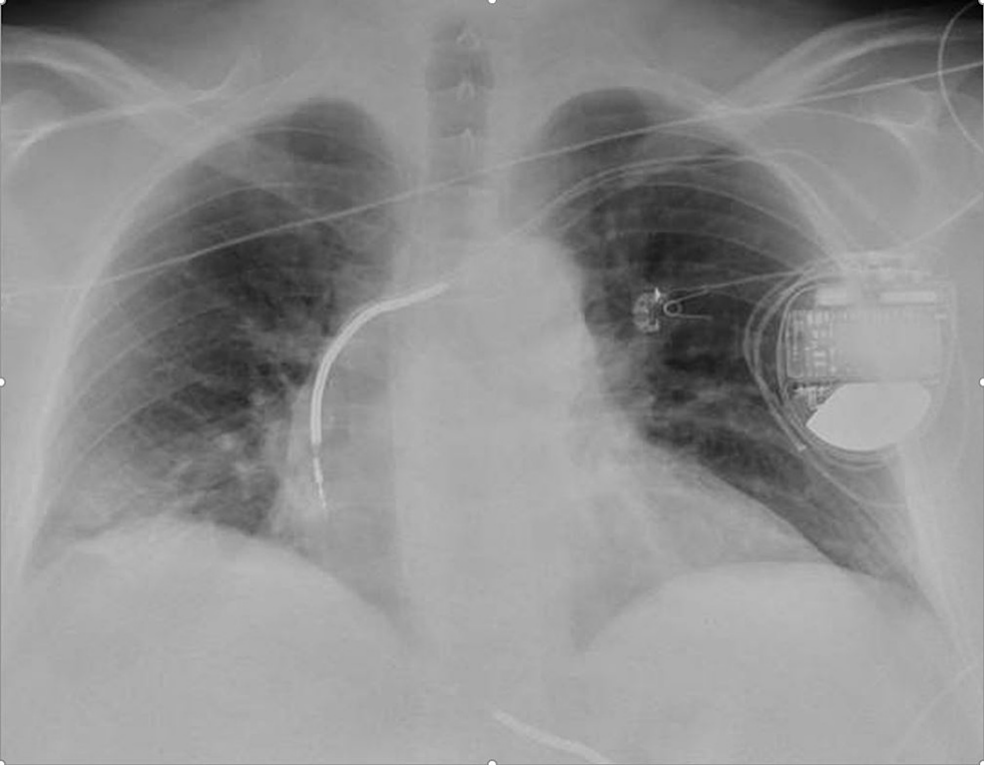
Chest x-ray showing elevated hemidiaphragm, which was confirmed to be chronic after comparison with prior x-rays. Paralysis was also ruled out with a negative sniff test.
